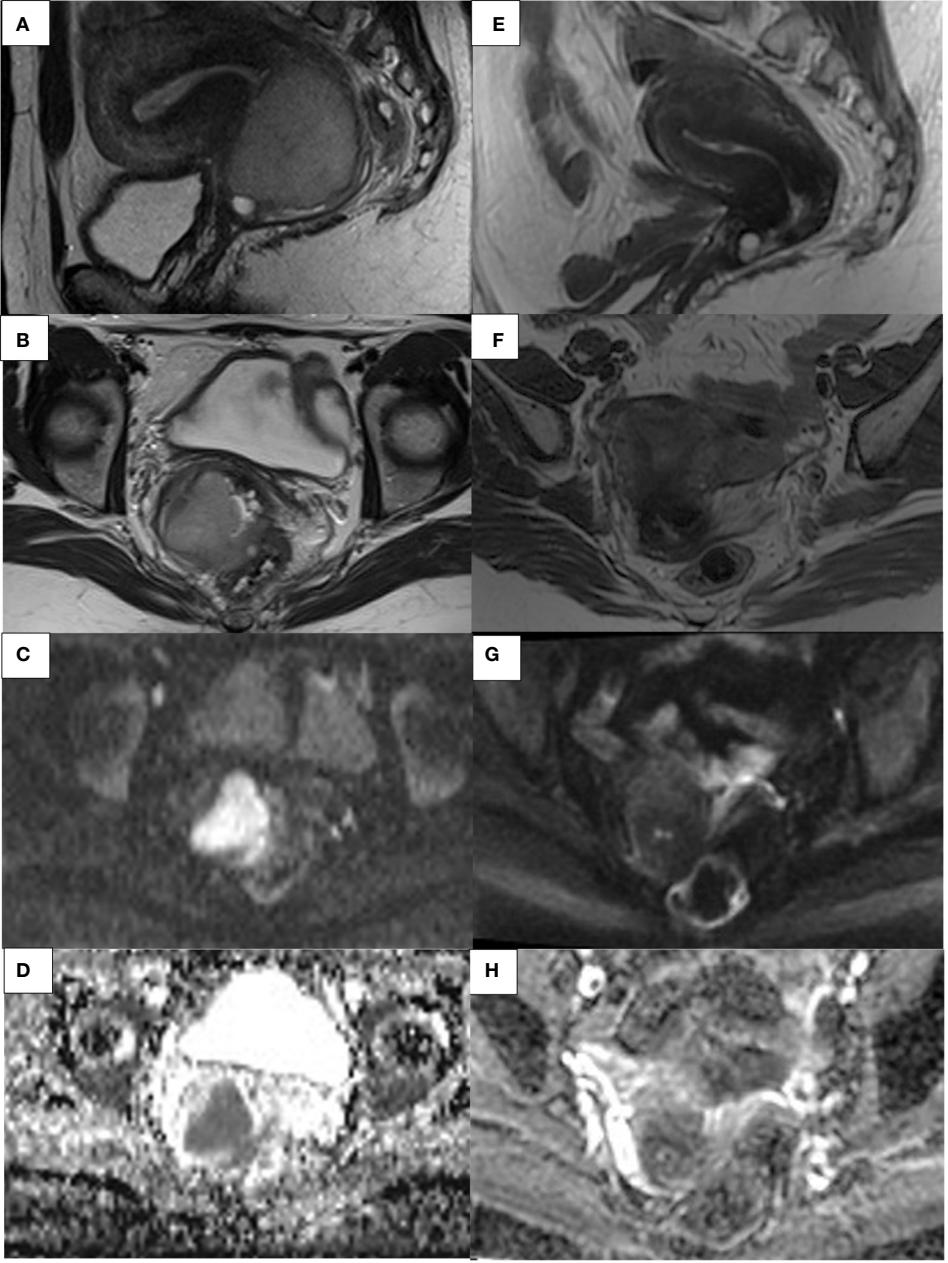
41- year-old woman. Sagittal and axial T2W (A, B) and axial DWI MR images with ADC map (C, D) show an invasive CC extending to the upper third of the vaginal canal and anterior rectal wall with focal parametrial infiltrations. Sagittal and axial T2W (E, F) and axial DWI MRI images with ADC map (G, H) shows a complete response after CCRT.
